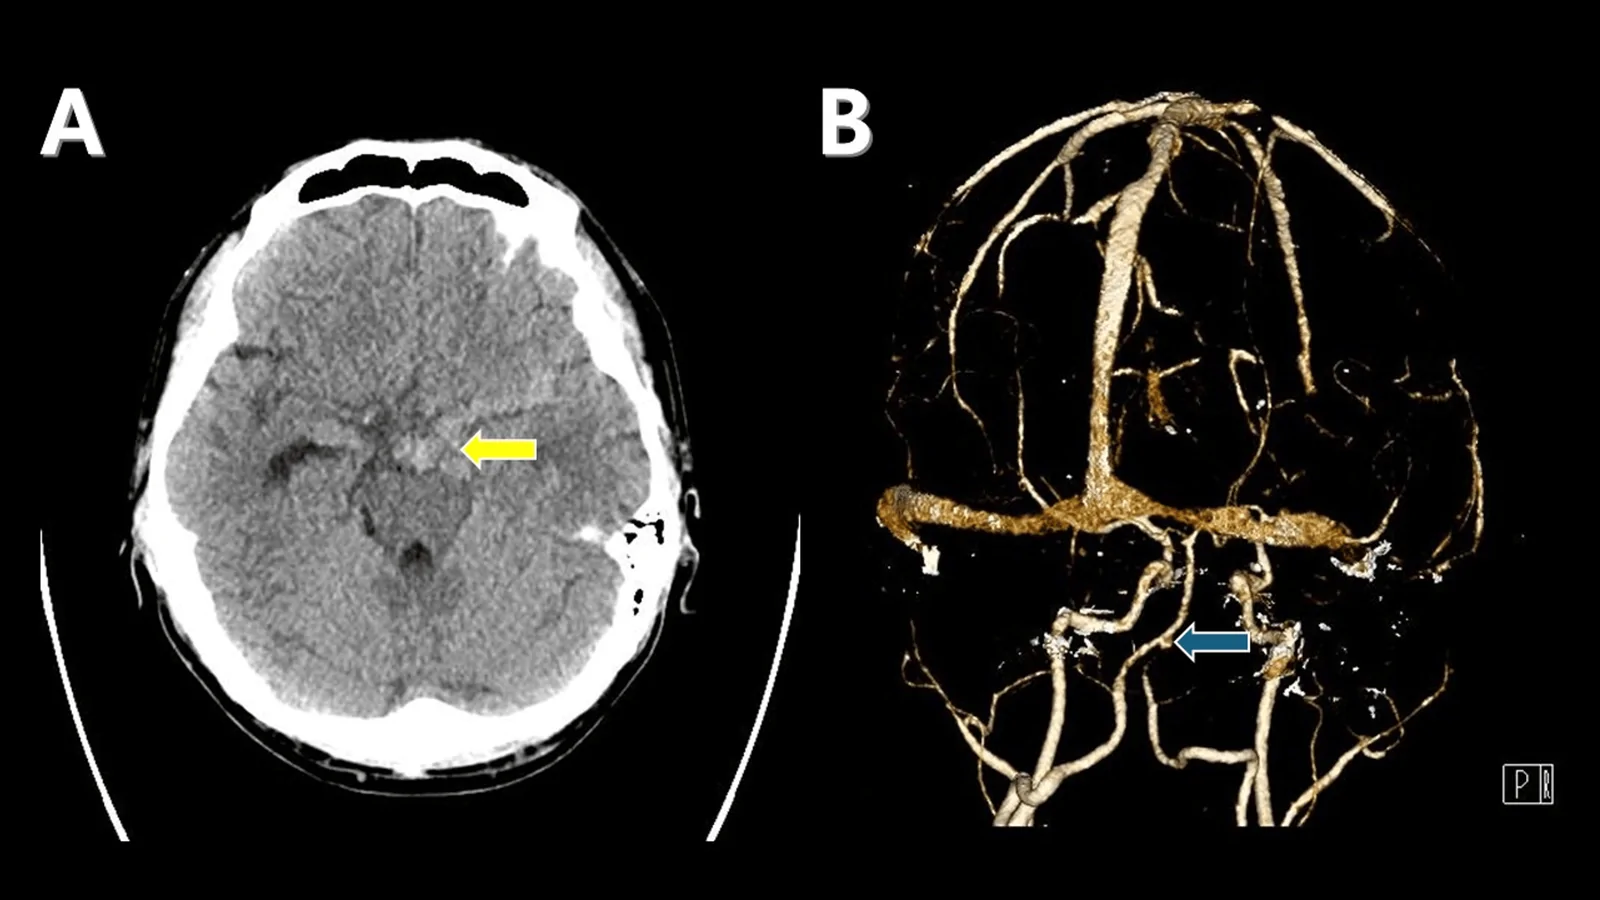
CTA showing a suspected cerebral aneurysm after a revisit to the emergency room for headache (A) A non-contrast brain CT scan performed upon presentation to the emergency room for headache reveals evidence of a subarachnoid hemorrhage (yellow arrow). (B) A 3D reconstructed CTA image shows a suspected aneurysm in an atypical location at the VB junction (blue arrow) CTA: computed tomography angiography; VB: vertebrobasilar
